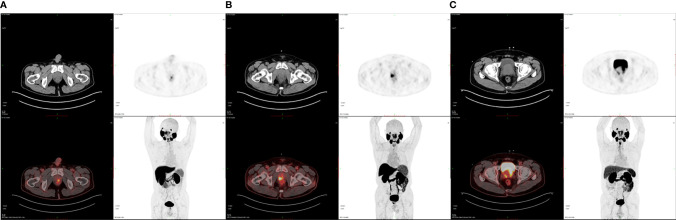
Representative ^18^F-DCFPyL PET/CT images of PCa patients at various risks. (A) SUVmax is 4.04 and the P/M ratio is 10.12 in a low-risk PCa patient. (B) SUVmax is 10.61 and the P/M ratio is 35.36 in an intermediate-risk PCa patient. (C) SUVmax is 30.94 and the P/M ratio is 91.26 in a high-risk PCa patient.
